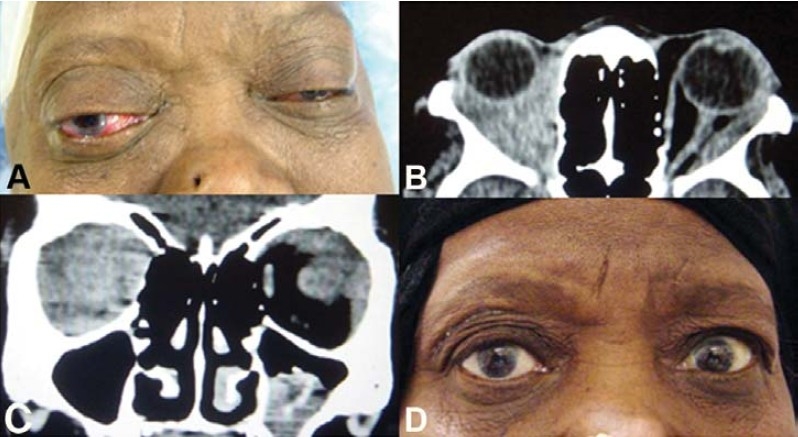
External photograph of a 60-year-old woman withhistory of several episodes ofrecurrent bilateral chemosis, restriction of extraocular motility and vision (A). CT-scan (axial and coronal) revealed bilateral infiltrative processes (B and C).Treatment with a course of corticosteroids and radiationtherapy resulted in resolution ofher symptoms (D).
